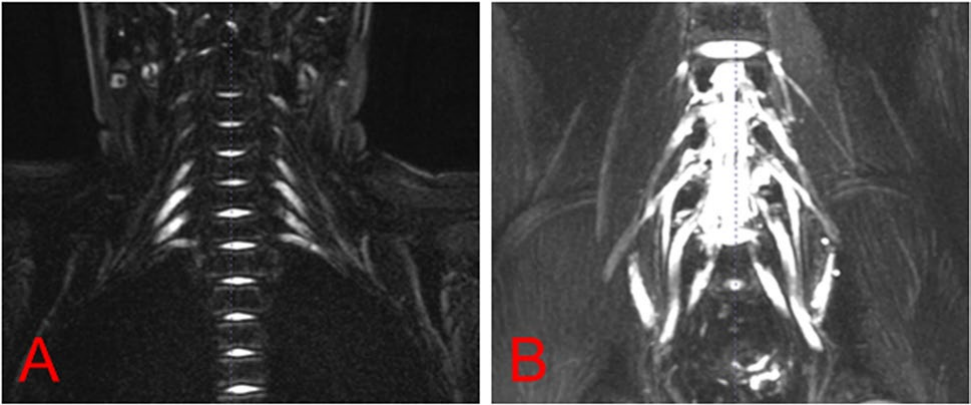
Neuroimaging. (A) MRI showed thickened bilateral brachial plexus nerves with T2 hyperintensity, without distortion or disruption. (B) revealed linear and patchy T1 and T2 hyperintensities in L1-L3 subcutaneous fat, with mild thickening and T2 hyperintensity in bilateral L4-5 nerve roots
